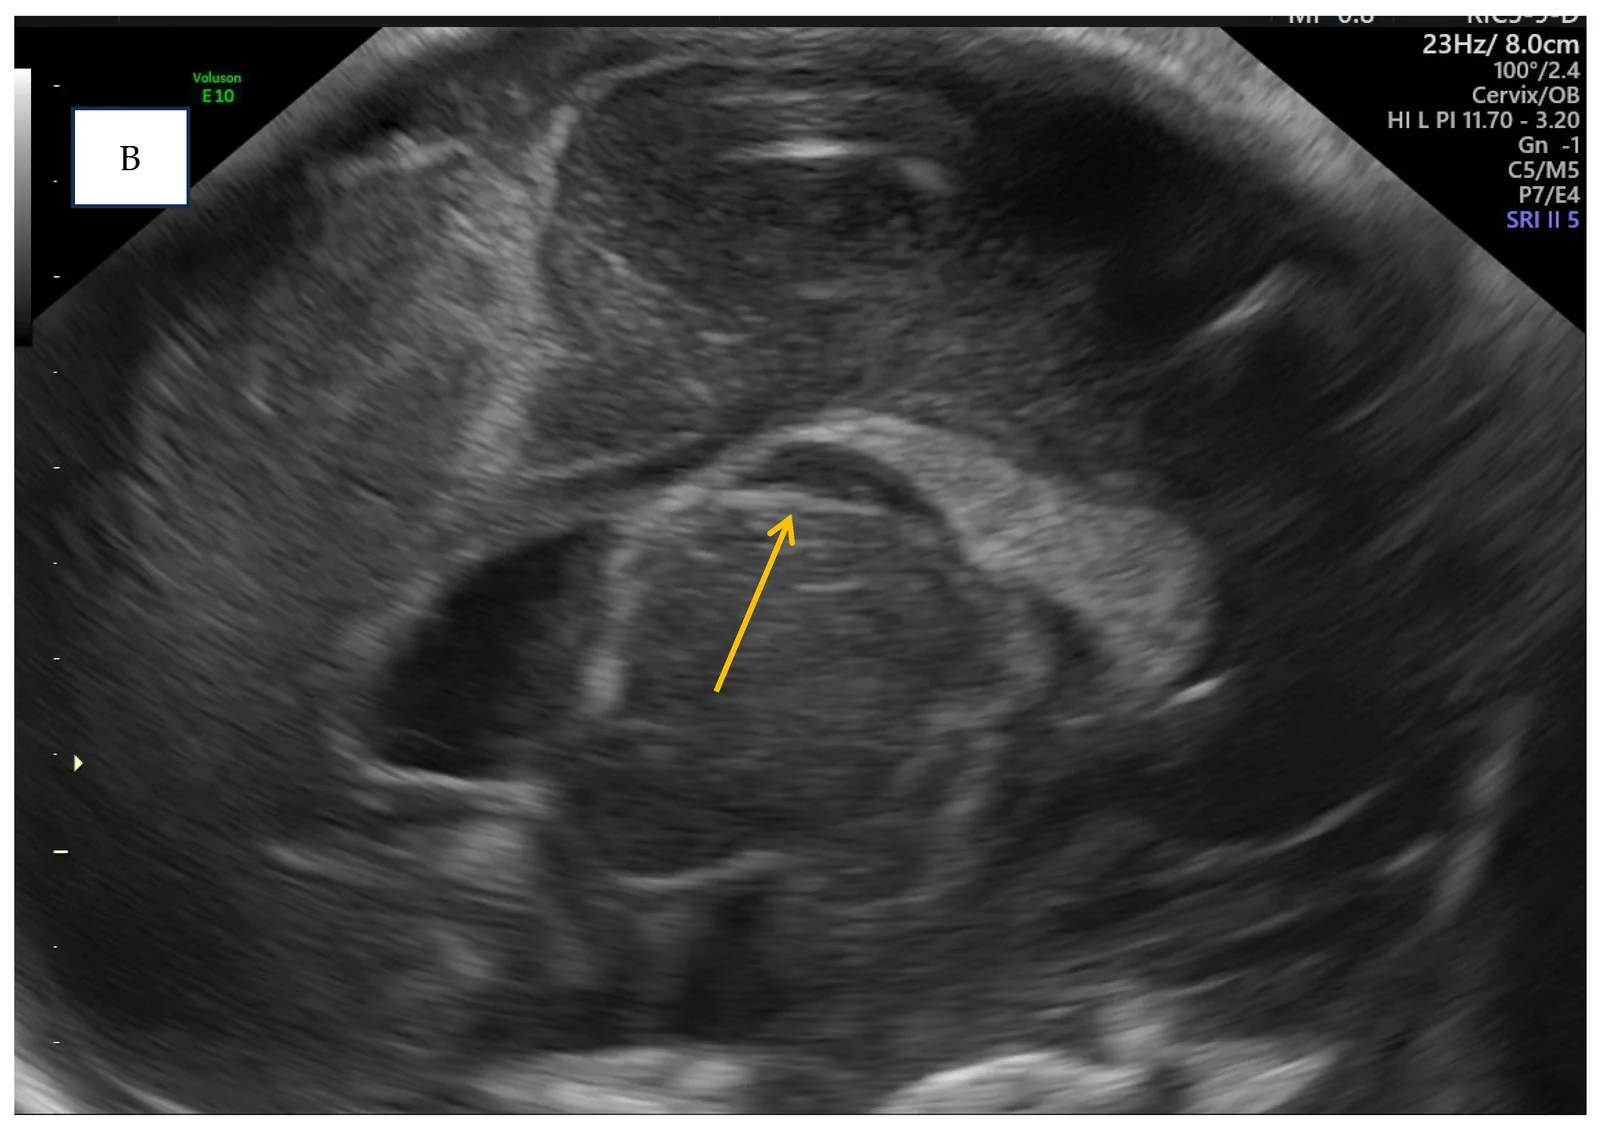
Representative transvaginal neurosonographic images of ganglionic eminence (GE) abnormalities at 20 weeks of gestation. (A) GE cavitation demonstrated in the coronal plane (A1) and parasagittal plane (A2). Note the characteristic hypoechoic cystic areas located anterior to the choroid plexus (indicator). (B) GE enlargement shown in the parasagittal plane with marked bulging of the GE into the lateral ventricle (arrow). GE enlargement is characterized by increased prominence and convex bulging of the GE into the lateral ventricle, with loss of its normal smooth ridge-like contour.
